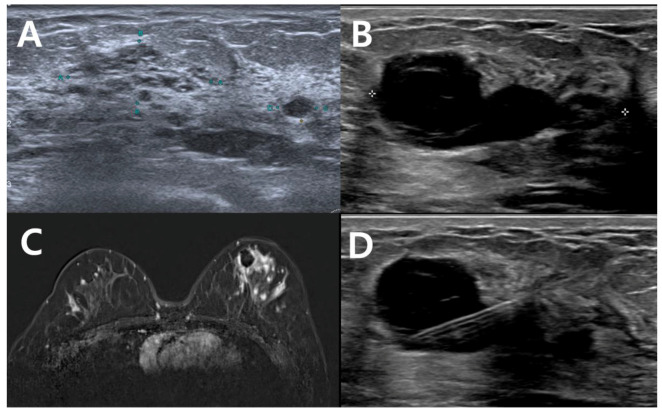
Radiologic findings of a representative case. (A) The initial non-mass lesion was excised by VABB and diagnosed with DCIS. (B) Second-look ultrasonography did not reveal residual tumor. (C) MRI revealed residual non-mass lesion surrounding post-VABB cavity. (D) The post-VABB site was localized with metallic wire and excised widely. Final pathology was a 4.2 cm sized DCIS.
